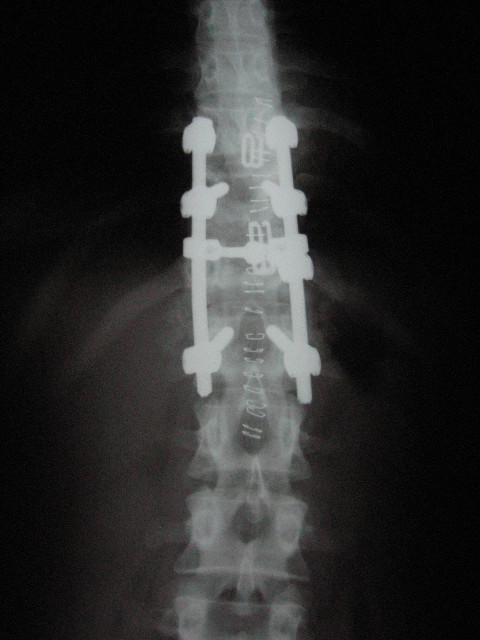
AP x-rays post-stabilization.
